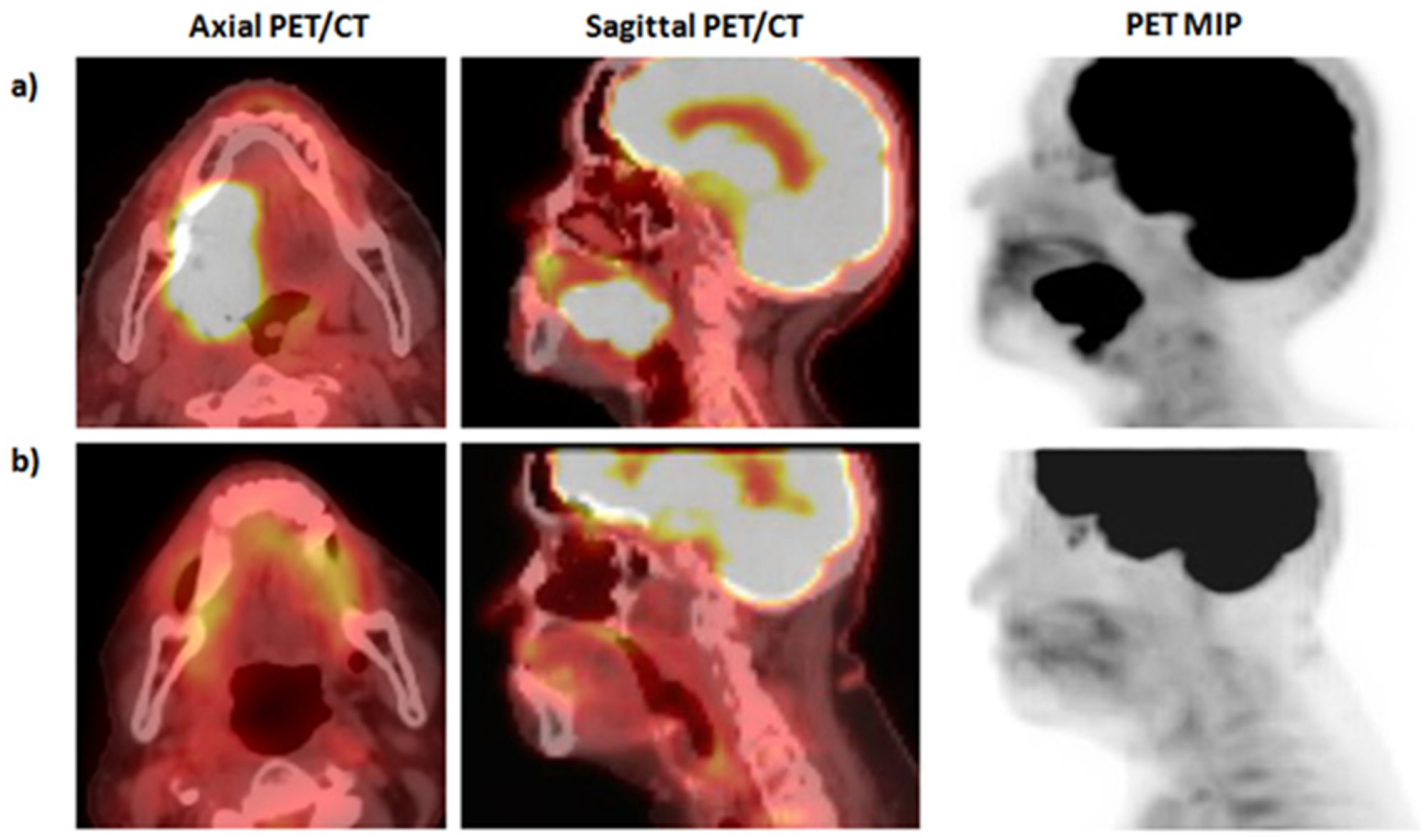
Pre- and post-therapy imaging for patient A *MIP: Fludeoxyglucose (^18^F) positron emission tomography (FDG-PET) maximum intensity projection (MIP). a) Pre-radiotherapy PET/CT demonstrates primary oral tongue SCC with SUV of 35.4 with FDG avid level Ib and II lymph nodes. b) Three month post-RT PET/CT demonstrates complete resolution of FDG avid malignancy.
